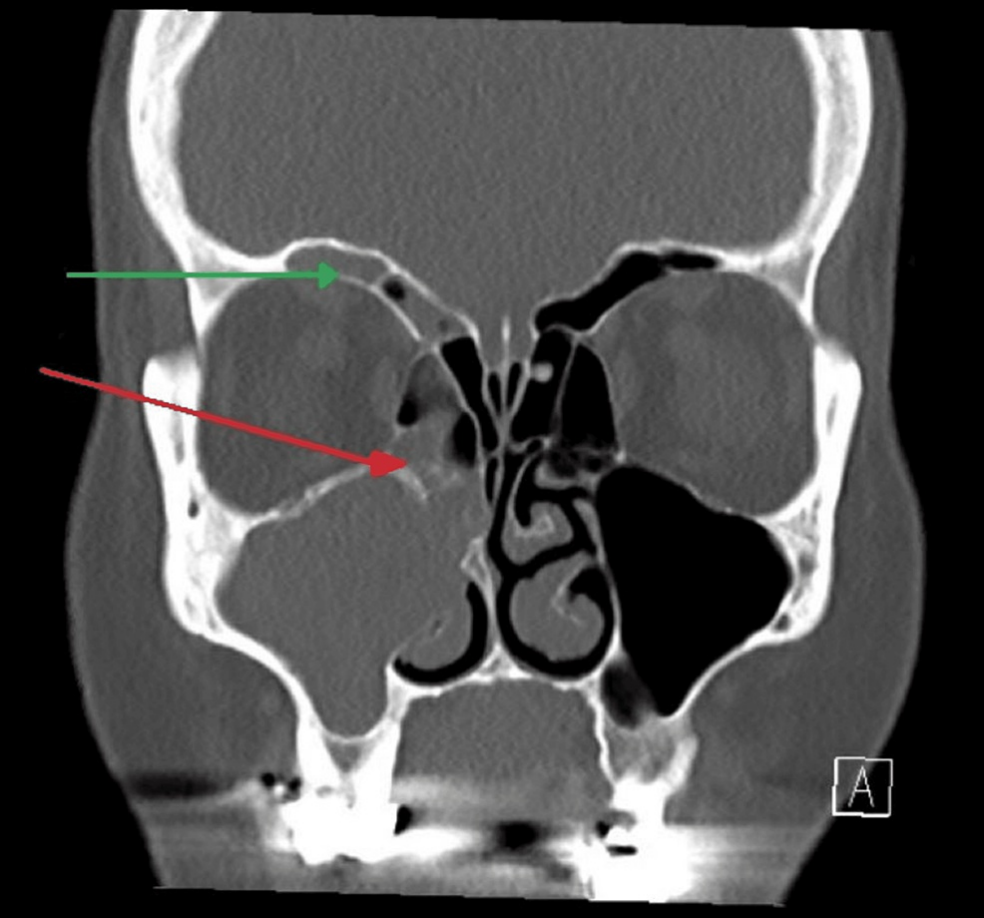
Computed tomography scan of paranasal sinus cavities Severe right maxillary (red arrow), anterior ethmoid, and frontal sinuses (green arrow) allergic fungal polypoid sinusitis with suspicious acute sinusitis.
